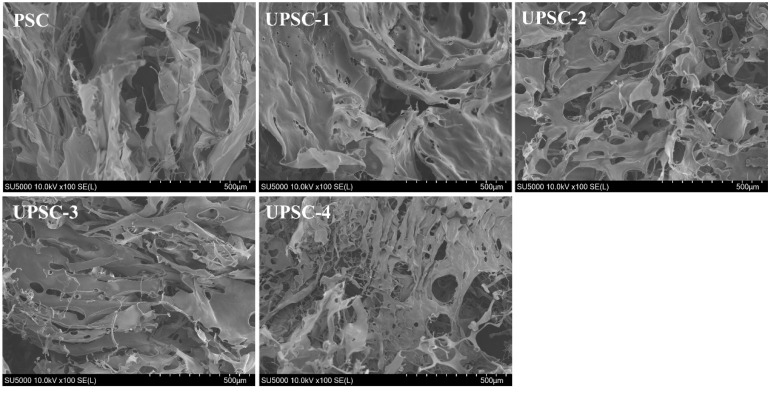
SEM images of pepsin-soluble collagen-I from albacore skin extracted at various ultrasonic powers, at 100× magnification.
